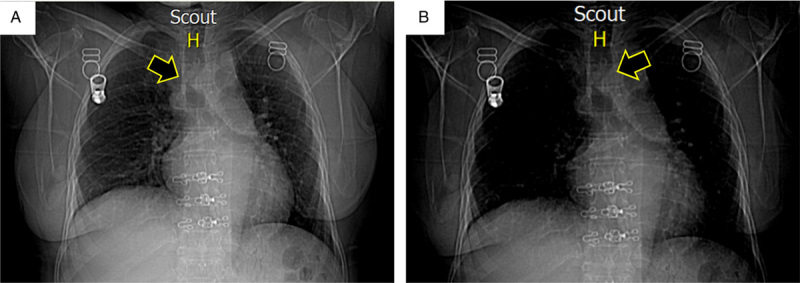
(A and B) A chest radiograph showing tracheal narrowing 2–3 cm above the carina (arrows).
